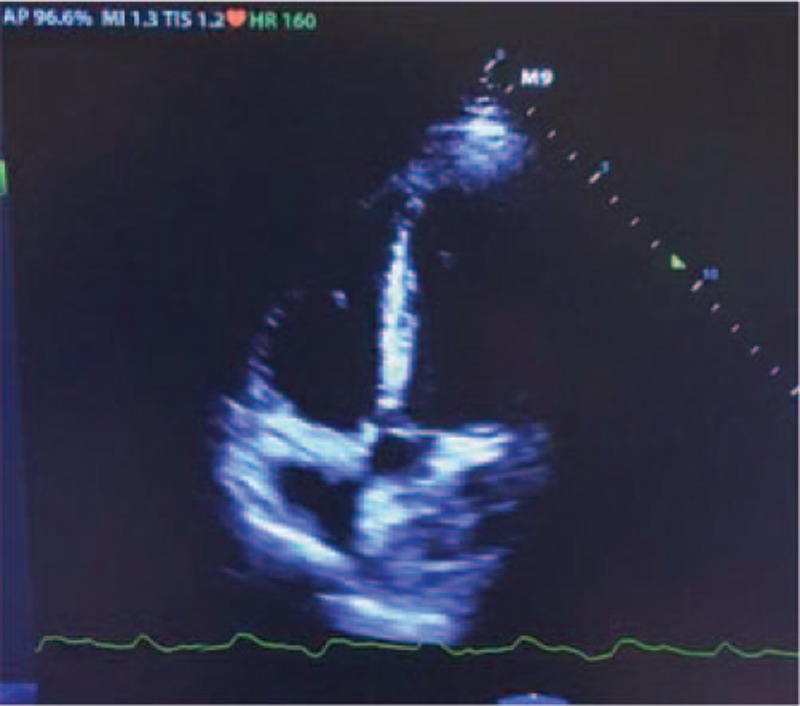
Echocardiography showed aortic valve hardly opened during left ventricular contraction.
